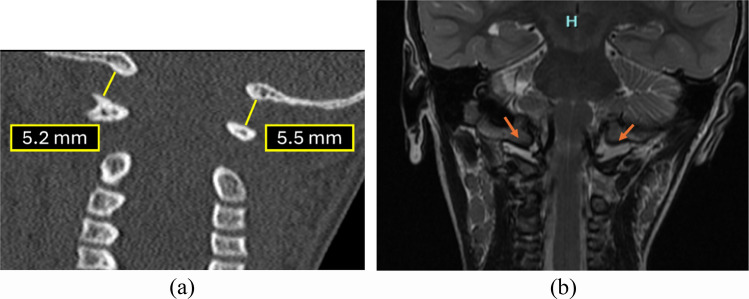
A 4-year-old child struck by a truck. (a) Coronal CT demonstrates an increased occipital condyle–C1 interval (normal <3.5 mm). (b) Corresponding coronal  T2-weighted MRI reveals fluid within the occipital condyle–C1 joint space (*orange arrows*), indicating ligamentous injury
